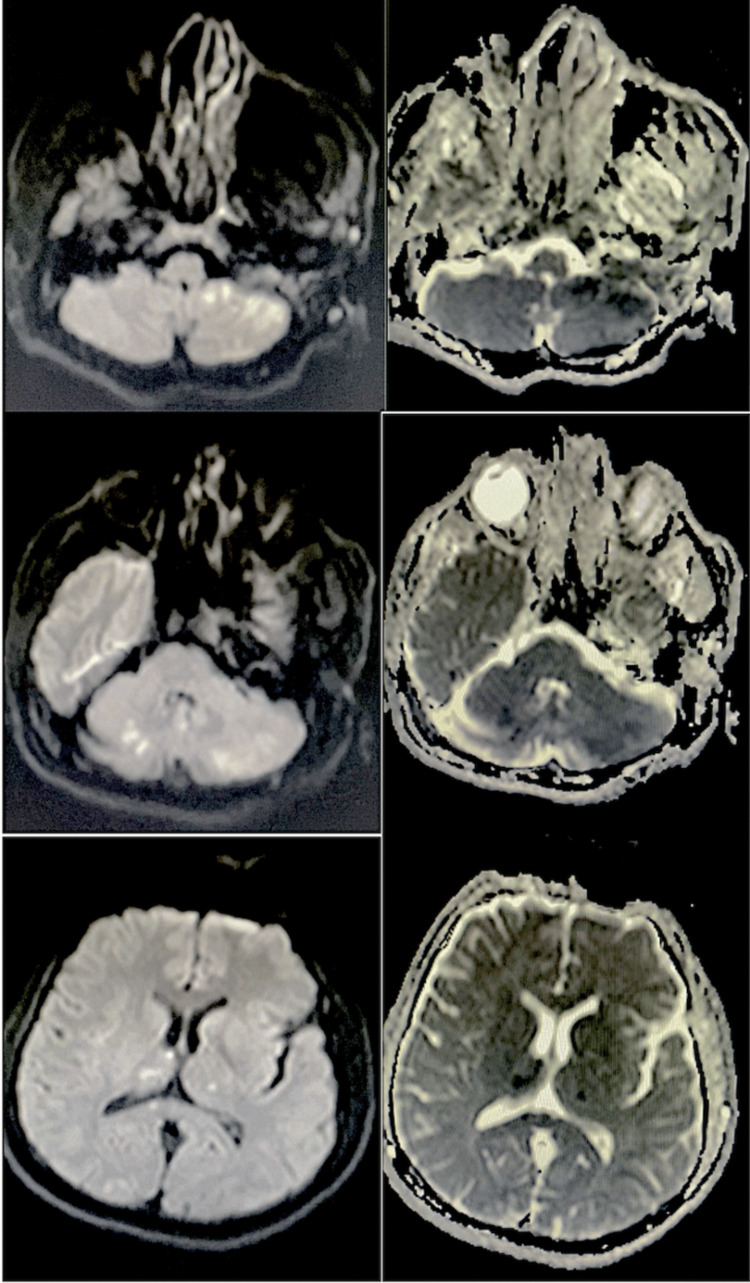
Magnetic resonance imaging (MRI) showed an acute infarction involving the bilateral cerebellar hemisphere and corpus callosum, thalamic area, and right occipital area
